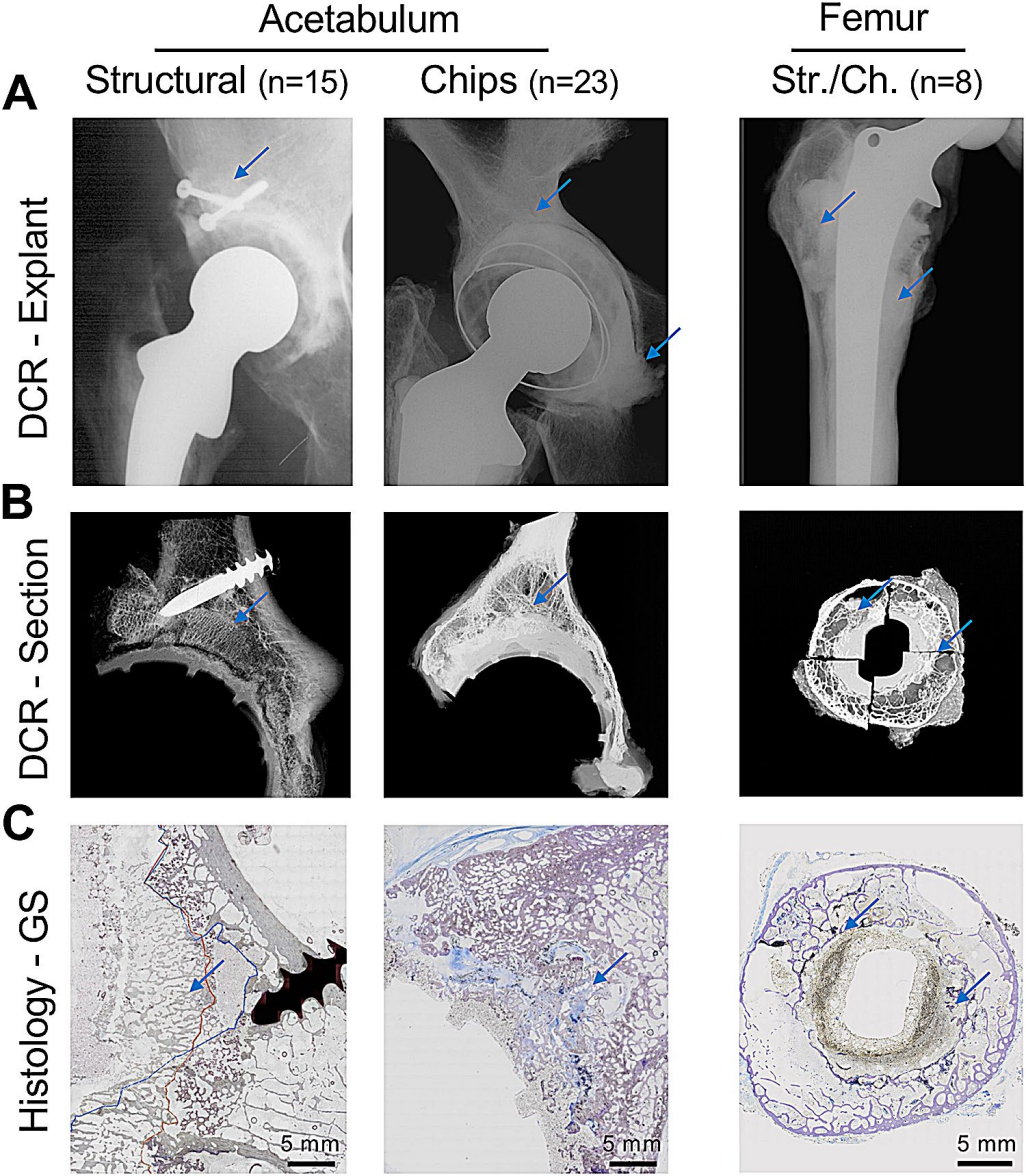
Imaging and processing of the hip explants in 46 cases (acetabulum and femur). (A) After explantation of the hip region, digital contact radiography (DCR) of the complete explant was performed first. Blue arrows indicate the allograft localization in accordance with available surgical reports. (B) Further processing included DCR of the cut sections, where the allograft region could already be identified (blue arrows). (C) Overview images of the ground sections (GS) reveal the bone microstructure of the host bone and the allograft. Blue arrows: allograft localization.
